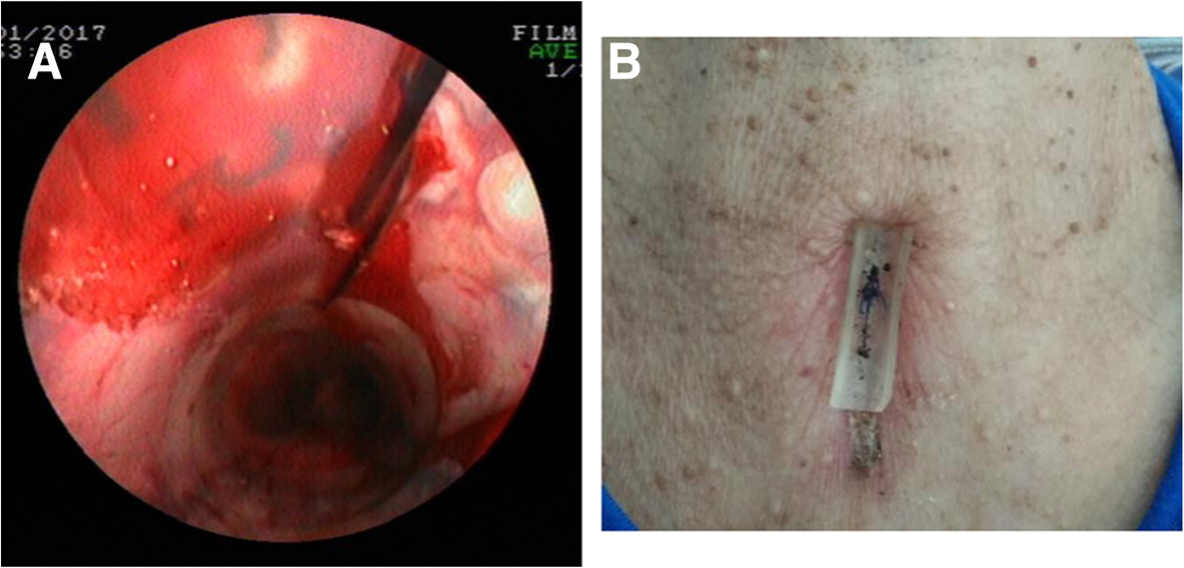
Images of the operation procedures. a: Suture fixation method for the tracheal stent. b: Postoperative CT showing the silicon pad on the skin at the puncture site (as the arrow indicates)
